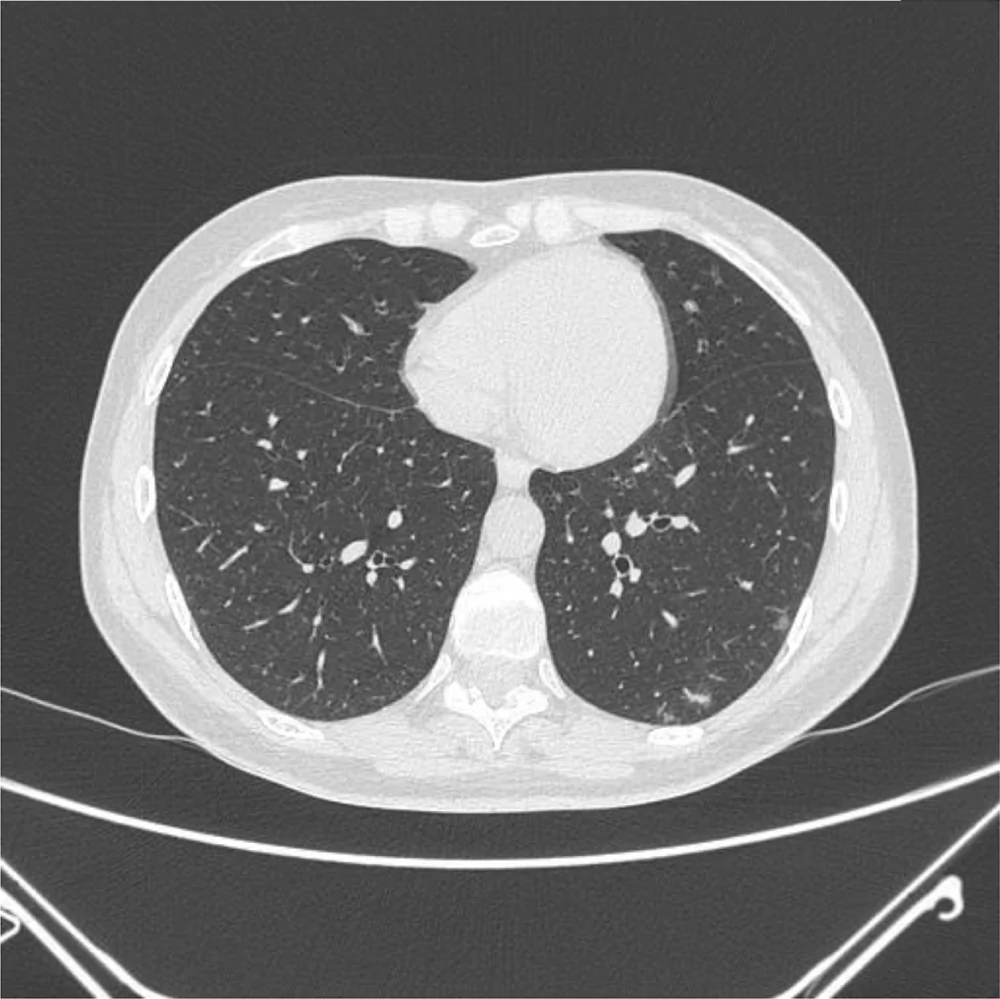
HRCT chest showing consolidation with air bronchogram and adjacent ground glass opacities in bilateral lung fields.
